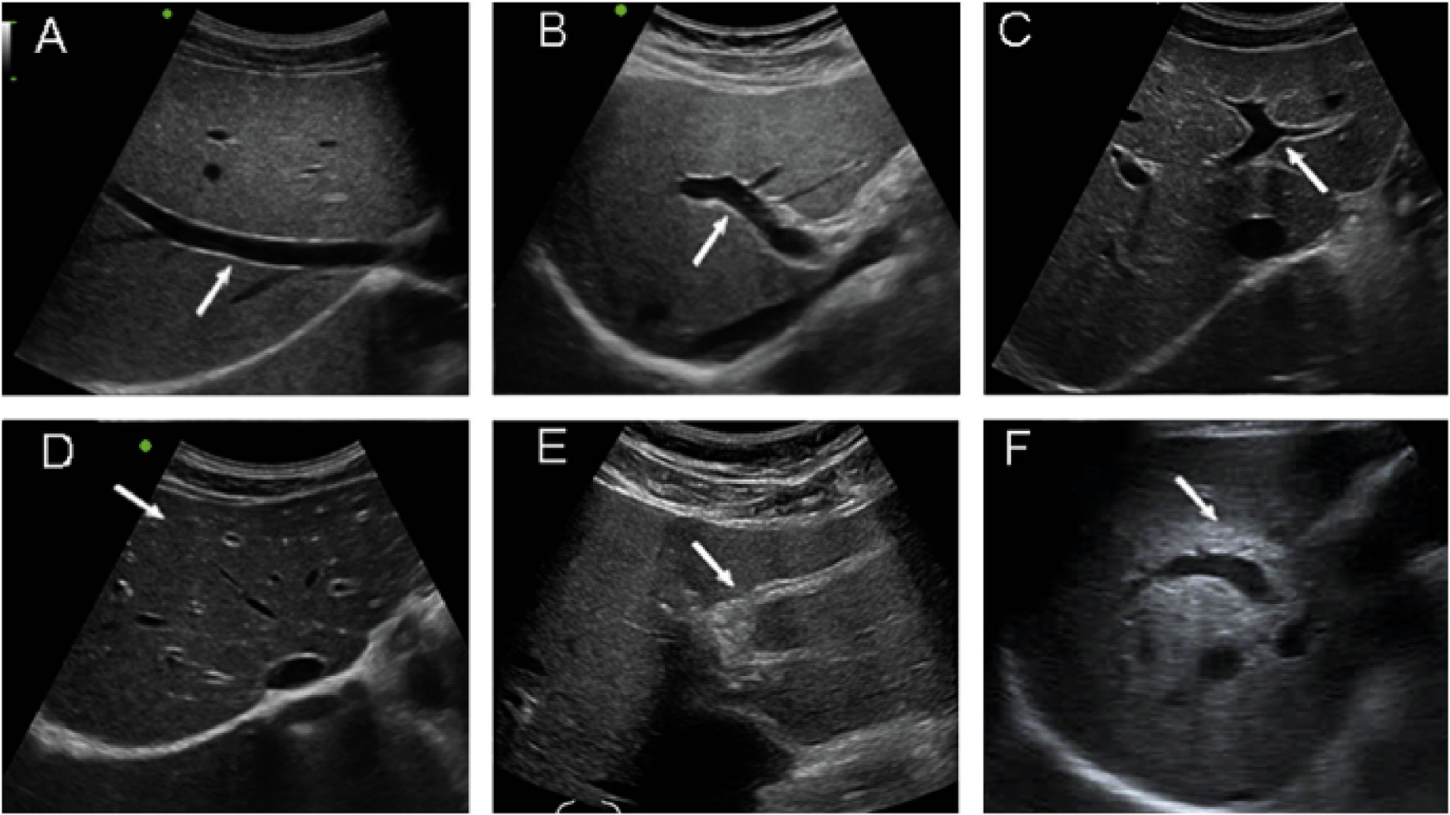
Ultrasound typing of periductal fibrosis (PDF). Normal echo pattern of the liver (A–C) with arrow pointing at (A) the right hepatic vein, (B) right portal vein and (C) left portal vein. (D) PDF+ shows a ‘starry sky appearance’, with bright echogenic dots along branches of portal vein (arrow); (E) PDF++ shows ring echoes around vessels in cross-section, pipe-stems parallel with portal vein (arrow) and (F) PDF+++ shows an echogenic ruff around the portal bifurcation and main stem; the main portal vein vessels show wall thickening (arrow). Figure reproduced with permission of the copyright owner.^6^
